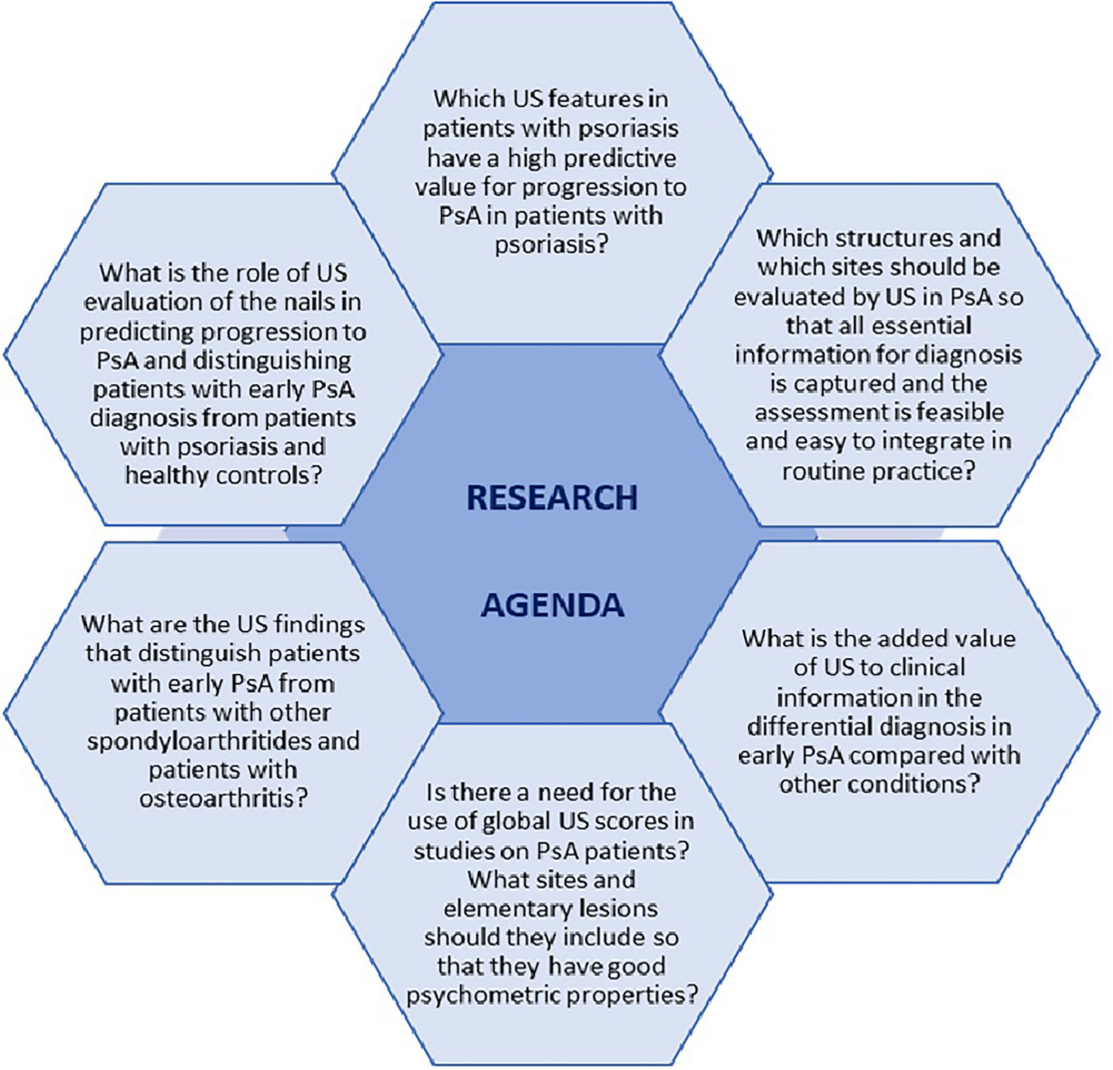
Research agenda for the use of ultrasound in early psoriatic arthritis.
